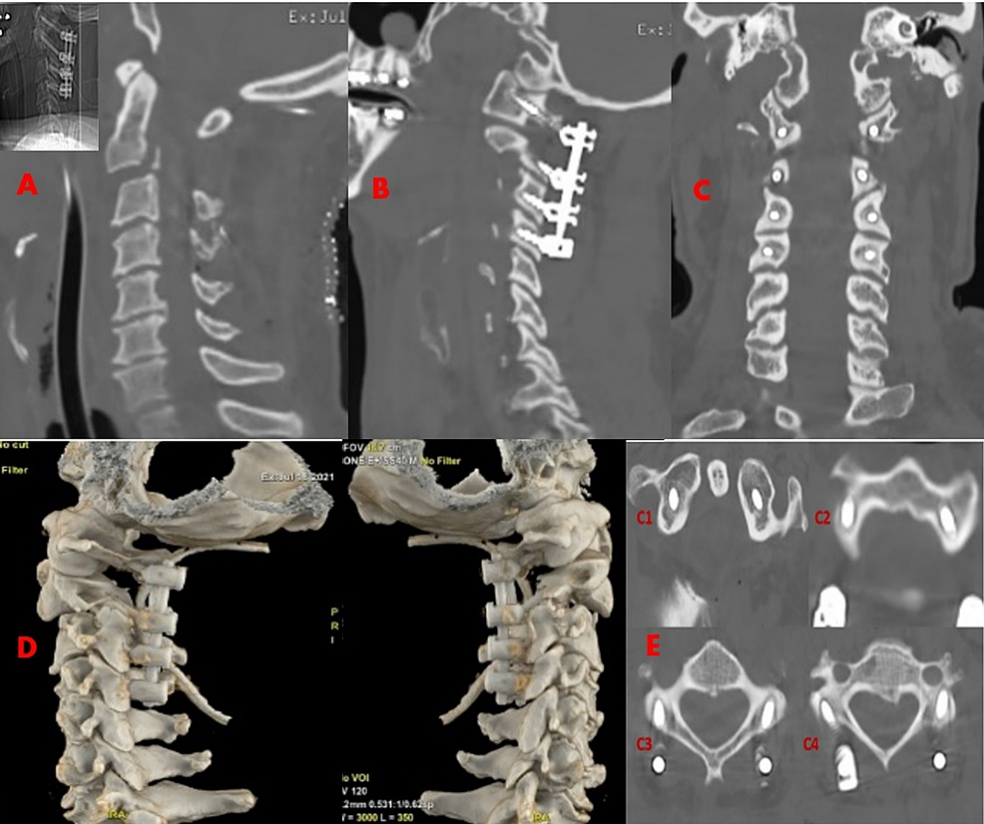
Postoperative cervical CT scan (bone) Sagittal and coronal (A-C), axial (C1-C4), and 3D (D-E).
